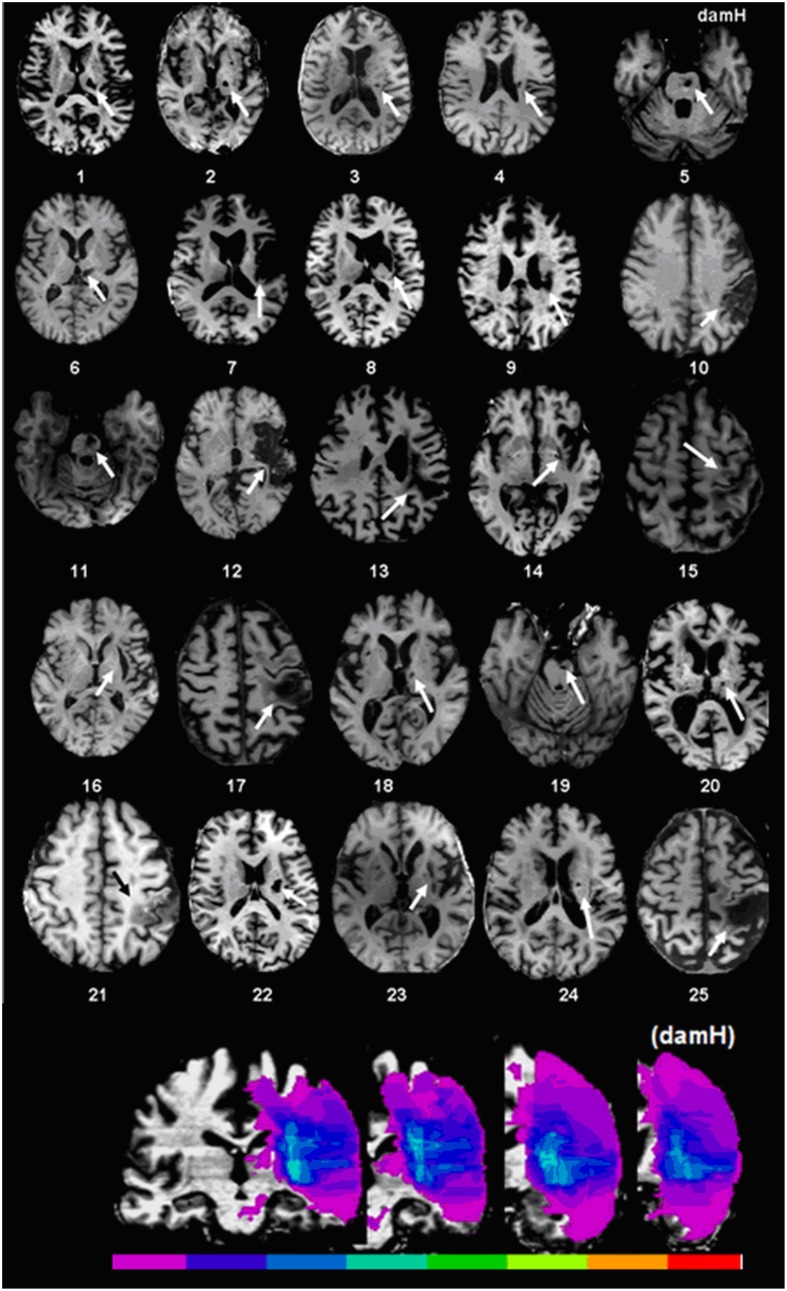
Stroke localization and overlap. Upper panel: T1 magnetic resonance imaging (MRI) at the level of the main stroke injury. Lower panel: Lesion overlap in stroke patients symbolized by the color scale. Purple represents the stroke area of a single patient, green represents localization shared by half of the patients, and red indicates localization shared by all of the patients. For patients with lesions on the right side of the brain, the 3D-T1 MRI was flipped. The map of lesion localization and overlap was created with MRIcro 1.4. DamH: damaged hemisphere.
